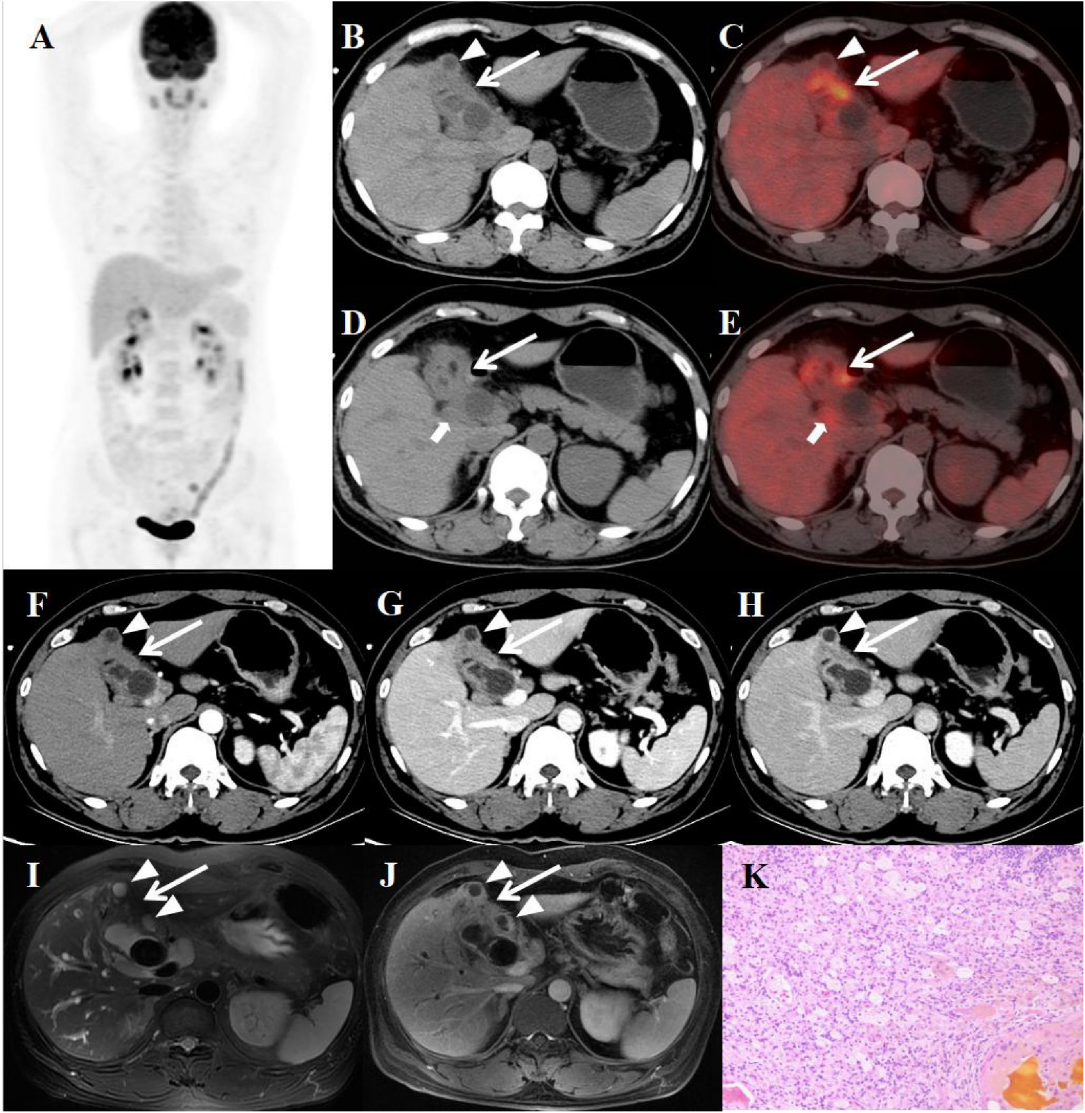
Female, 49 years old. (A) (whole body MIP), (B–E) (Axial CT and fused axial PET-CT), (F–H) (axial, multiphasic contrast-enhanced CT), (I) (axial, MRI-T2WI), (J) (axial, enhanced-MRI-delayed phase), and (K) (H-E × 40). 18F-FDG PET/CT Imaging Findings: Thickening of the gallbladder wall with indistinct boundaries from the liver, accompanied by increased FDG uptake with a SUVmax of 7.5 (B–E, arrow
